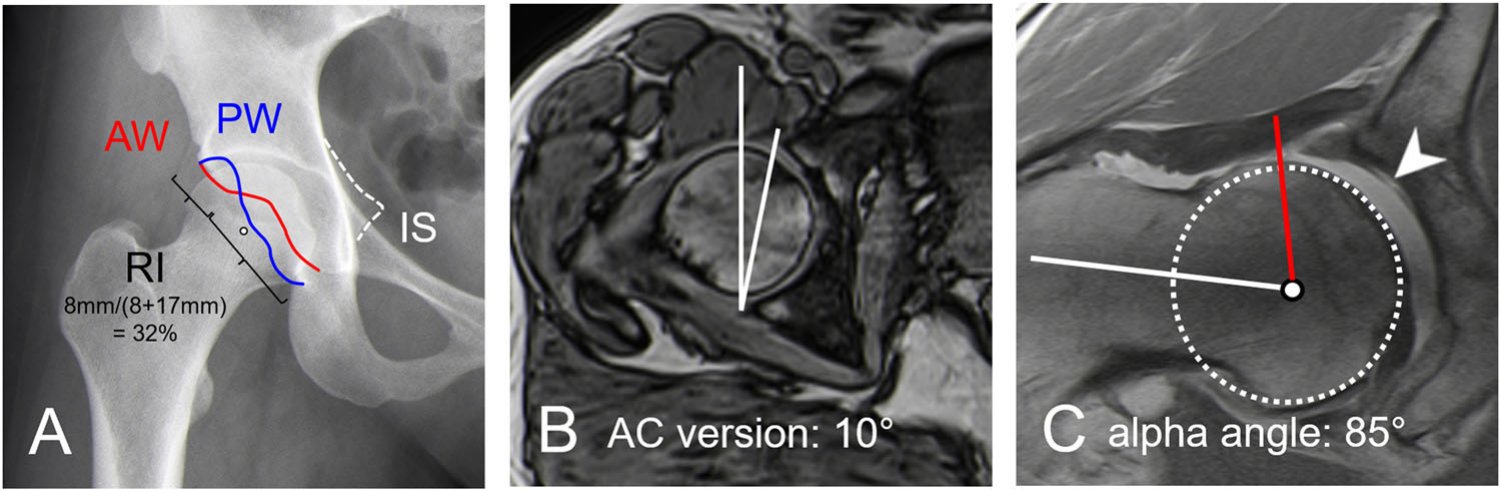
A AP pelvic radiograph shows acetabular retroversion with a positive cross-over sign with an anterior wall (AW) projecting lateral to the posterior wall (PW), the extension of the retroverted part relative to the entire acetabulum is reflected by the retroversion index (RI of 32%); positive posterior wall sign with a projection of the posterior wall (PW) medial to the femoral head center and positive ischial spine sign with the projection of the ischial spine (IS) into the pelvic inlet. B Measurement of the acetabular version of 10° at the level of the center of the femoral head is shown on axial T1-w volume interpolated breath-hold examination DIXON sequence. C Measurement of alpha angle of 85° on a radial proton-density weighted turbo spin echo image indicating the presence of a cam deformity, note delamination of the acetabular cartilage (arrowhead)
